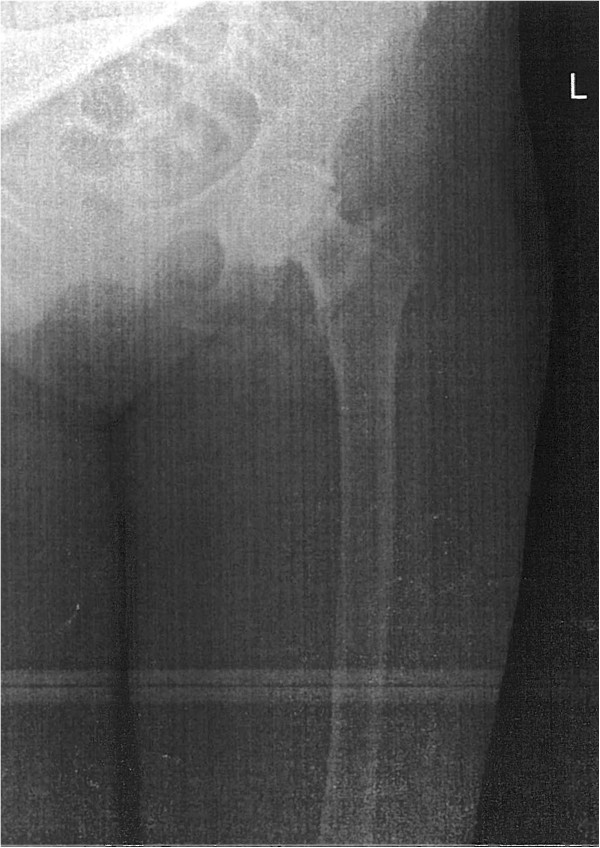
Emergency X-ray showing an Evans III pertrochanteric fracture and Kellgren-Lawrence III hip osteoarthritis.
